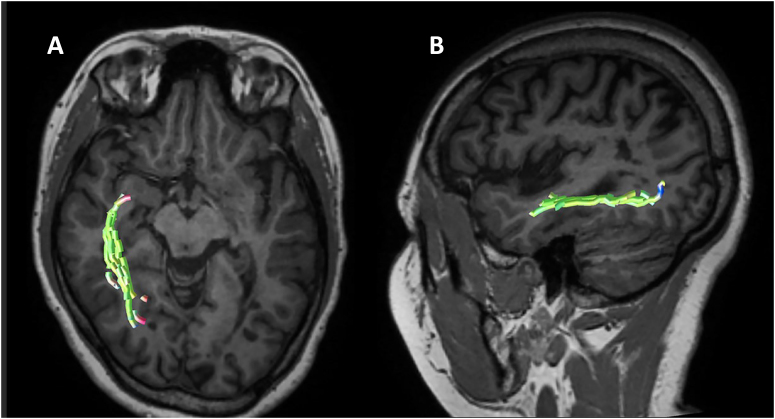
Inferior longitudinal fasciculus. Image A) Diffusion tensor magnetic resonance imaging; axial view; and B) Diffusion tensor magnetic resonance imaging; sagittal view. Courtesy of Diogo Fragoso Cardoso.
